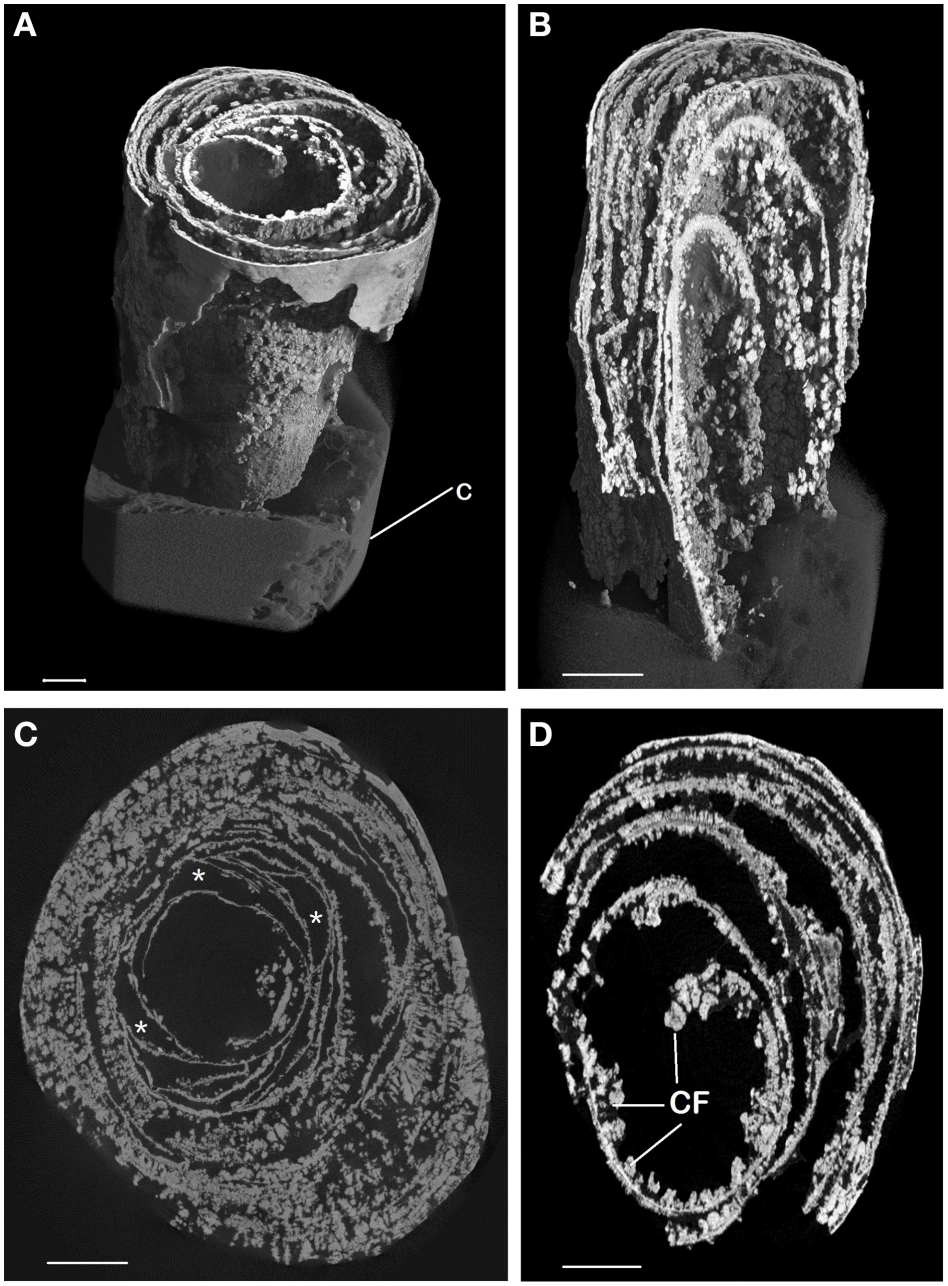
Micro CT scan of CaCO_3_ stalactite. (A) General habitus of CT scan of CaCO_3_ stalactite that contained nematodes. The area shown is near the tip of the soda straw at the bottom of the stalactite away from the ceiling. The bent laminae are clearly visible as is the knob like outgrowths on them. Cotton (C) was used to stabilize the stalactite for scanning. (B) Longitudinal section inside the lower part of image (A). The lamellar built of the stalactite and the outgrowths are plainly visible. (C,D) Cross sections of the stalactite in an area close to the ceiling (C) and near the end (D). Outgrowths can become very dominant and the laminae can form complete or partially enclosed spaces (^*^) within the inner stalactite. Scale bars: (A) 4 cm, (B): 0.1 cm, (C): 7cm, (D): 5 cm.
